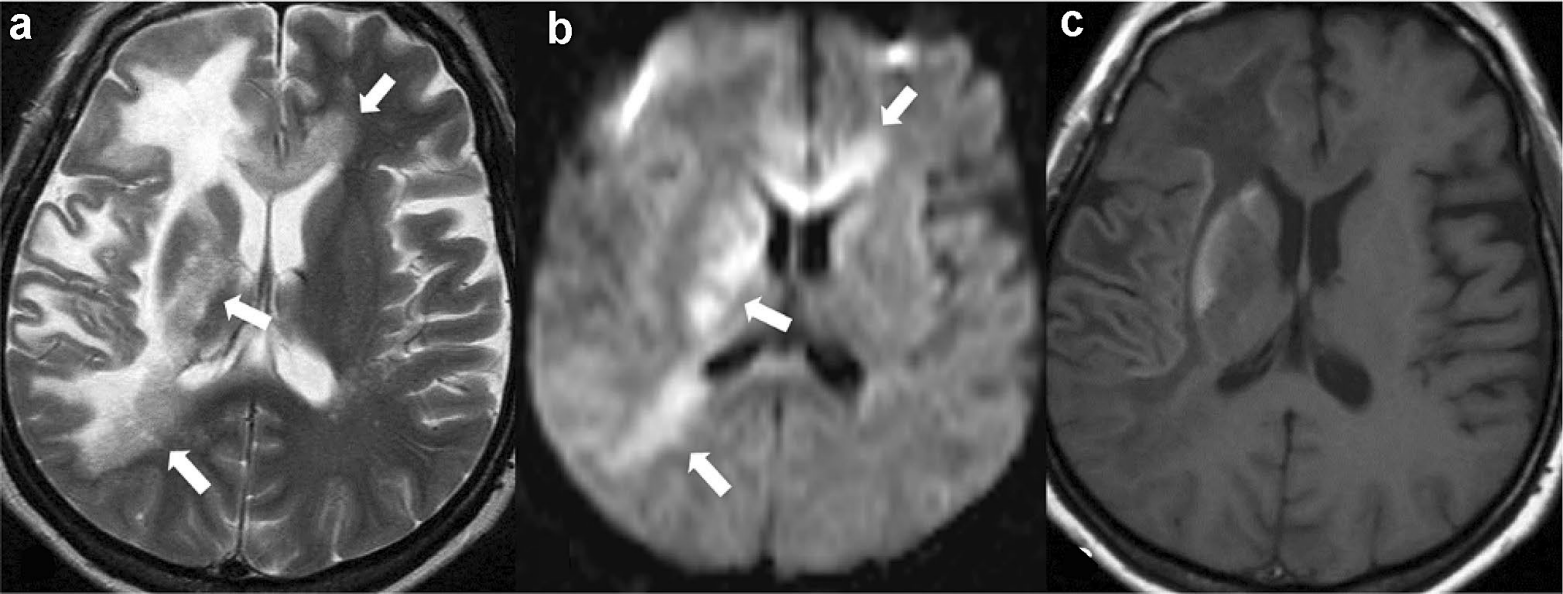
Heterogeneous demyelination in a patient with HIV-related progressive multifocal leukoencephalopathy. On T2-weighted image (a), the older demyelinated lesion shows homogenous hyperintensity, while its medial margin, frontline (white arrows), shows heterogeneous pale hyperintensity with numerous punctate hyperintensities (milky way appearance). On diffusion-weighted image (b), the hyperintensity area (DW hyperintensity rim) corresponding to the milky way appearing area is shown. On T1-weighted image (c), hyperintensity is seen in the right head of the caudate nucleus, putamen, and subcortical area. They are adjusted to a large confluent demyelinated area showing homogenous hyperintensity on T2-weighted image (a) and hypointensity on T1-weighted image (c). The image a and b were reprinted from [9] with permission
